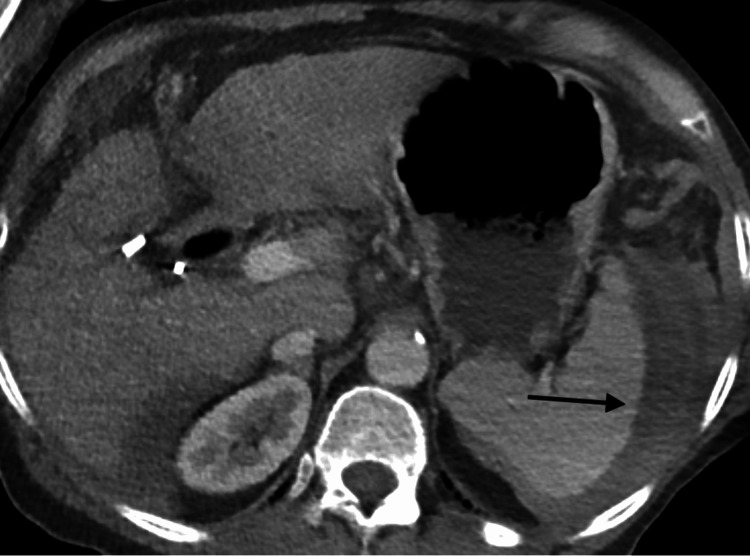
CT scan with IV contrast of the abdomen and pelvis demonstrating a splenic laceration The black arrow is pointing towards the area of hemorrhage from an inferior pole splenic laceration. The fluid about the spleen is hyperdense, and additionally, there is a focus of contrast just inferior to the inferior pole with associated hemoperitoneum.
